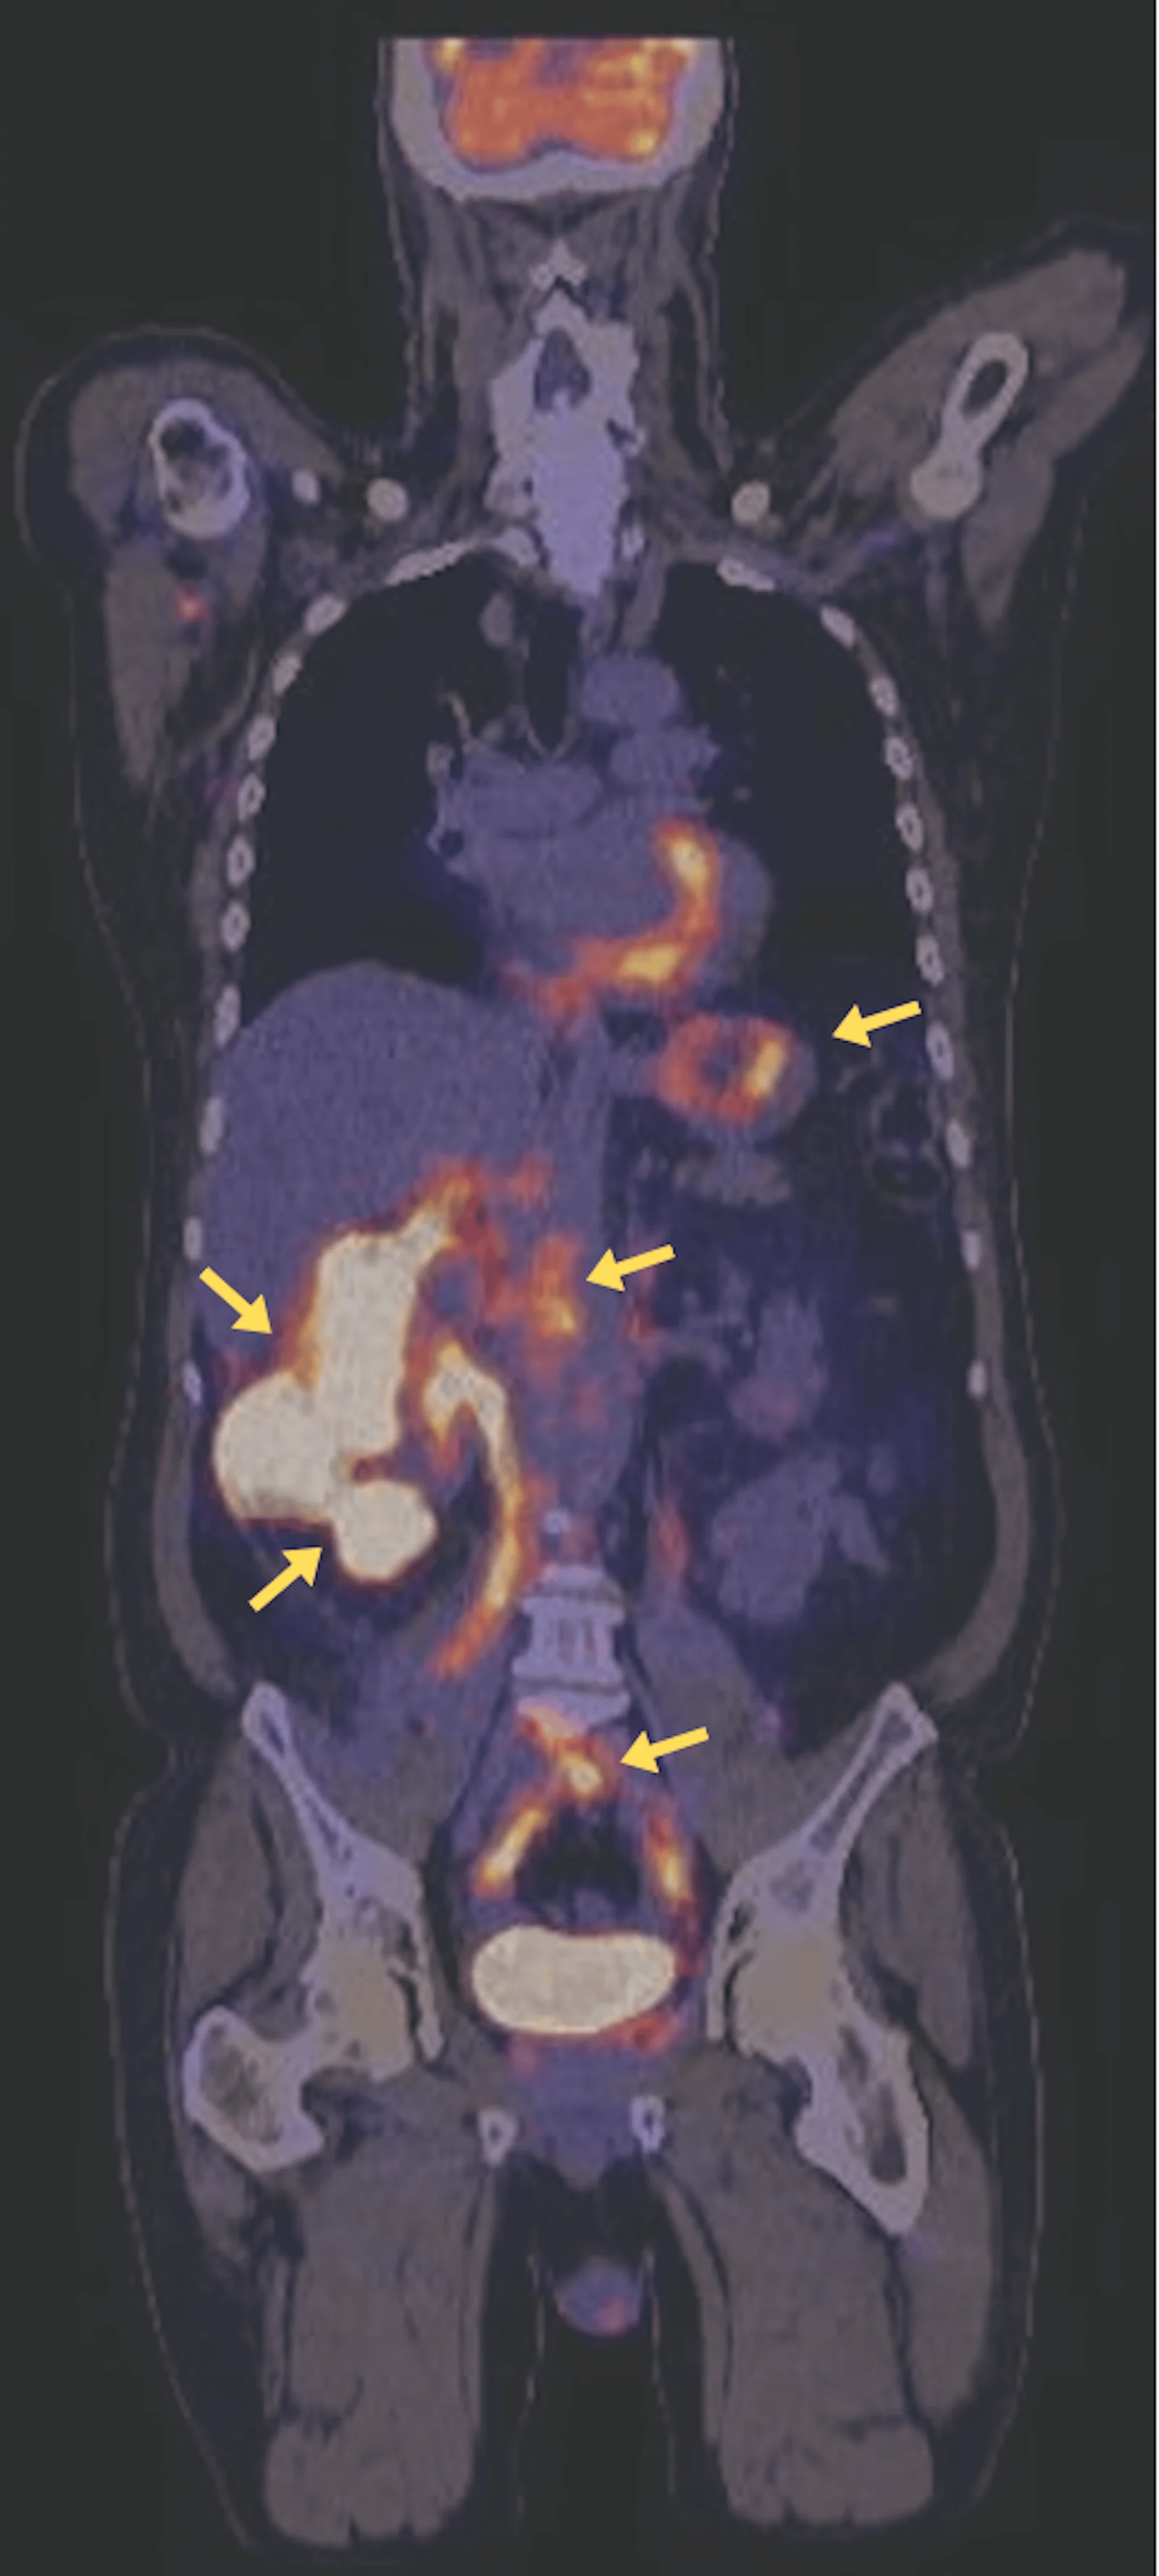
PET/CT with 18F-FDG Tumor viability due to lymphoma with nodal involvement above and below the diaphragm, as well as gastric, right crus, ipsilateral kidney, and mesorectal fascia involvement. PET/CT: positron emission tomography/computed tomography; 18F-FDG: fluorodeoxyglucose F-18
